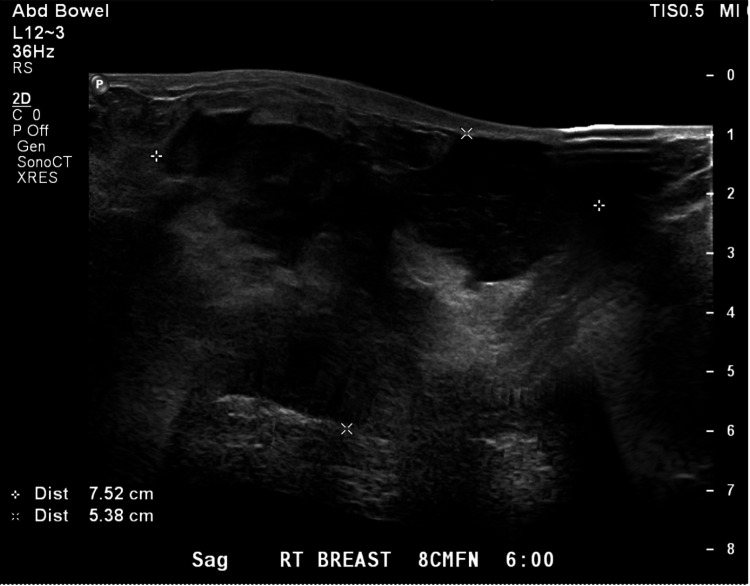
Breast ultrasound image (II) Breast ultrasound showing 10.1 x 5.4 x 7.5 cm partially solid mass with concern for possible malignancy.
